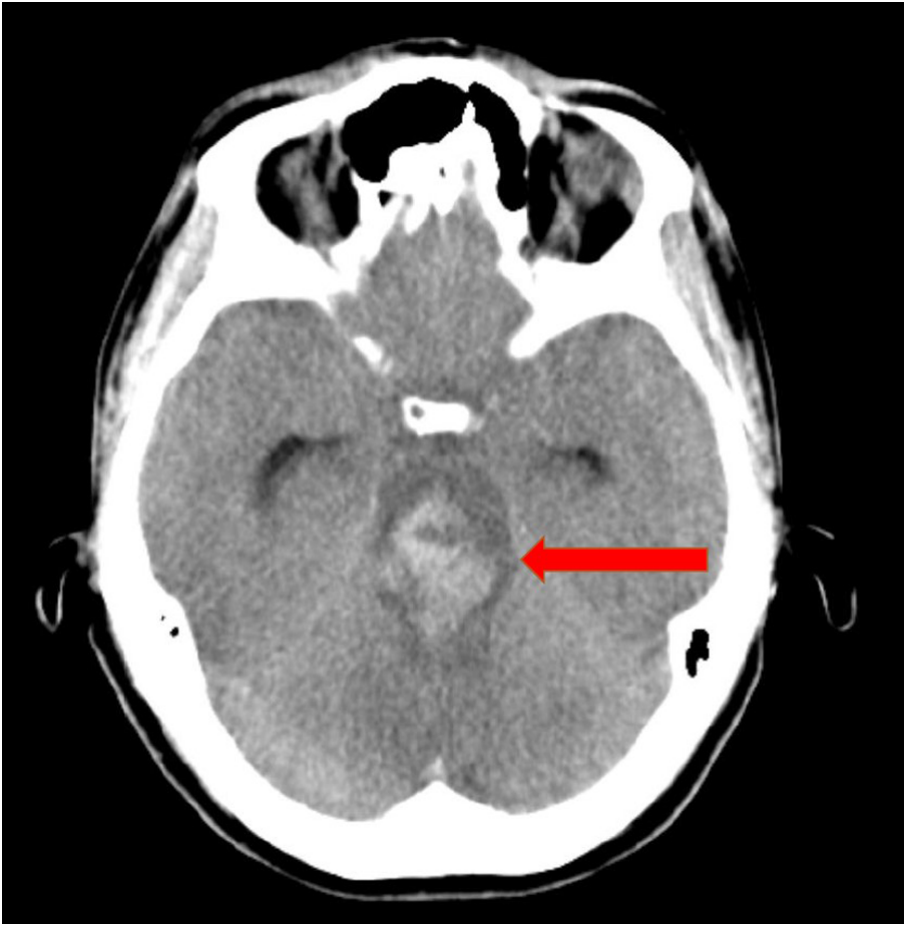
CT angiography shows extensive subarachnoid hemorrhage in the area of the mesencephalon and pons.
